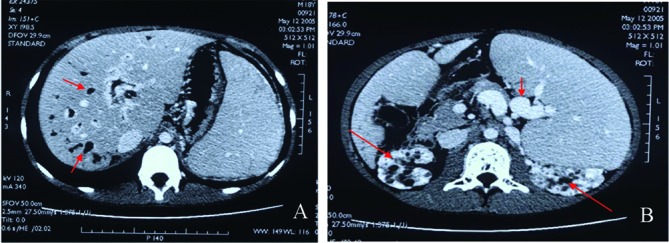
A: Abdominal enhanced CT scan, dilated intra-hepatic bile ducts (red arrow) and hepatosplenomegaly; B: Splenomegaly, circuitous spleen vein (short red arrow), and medullary sponge kidney (long red arrow).
